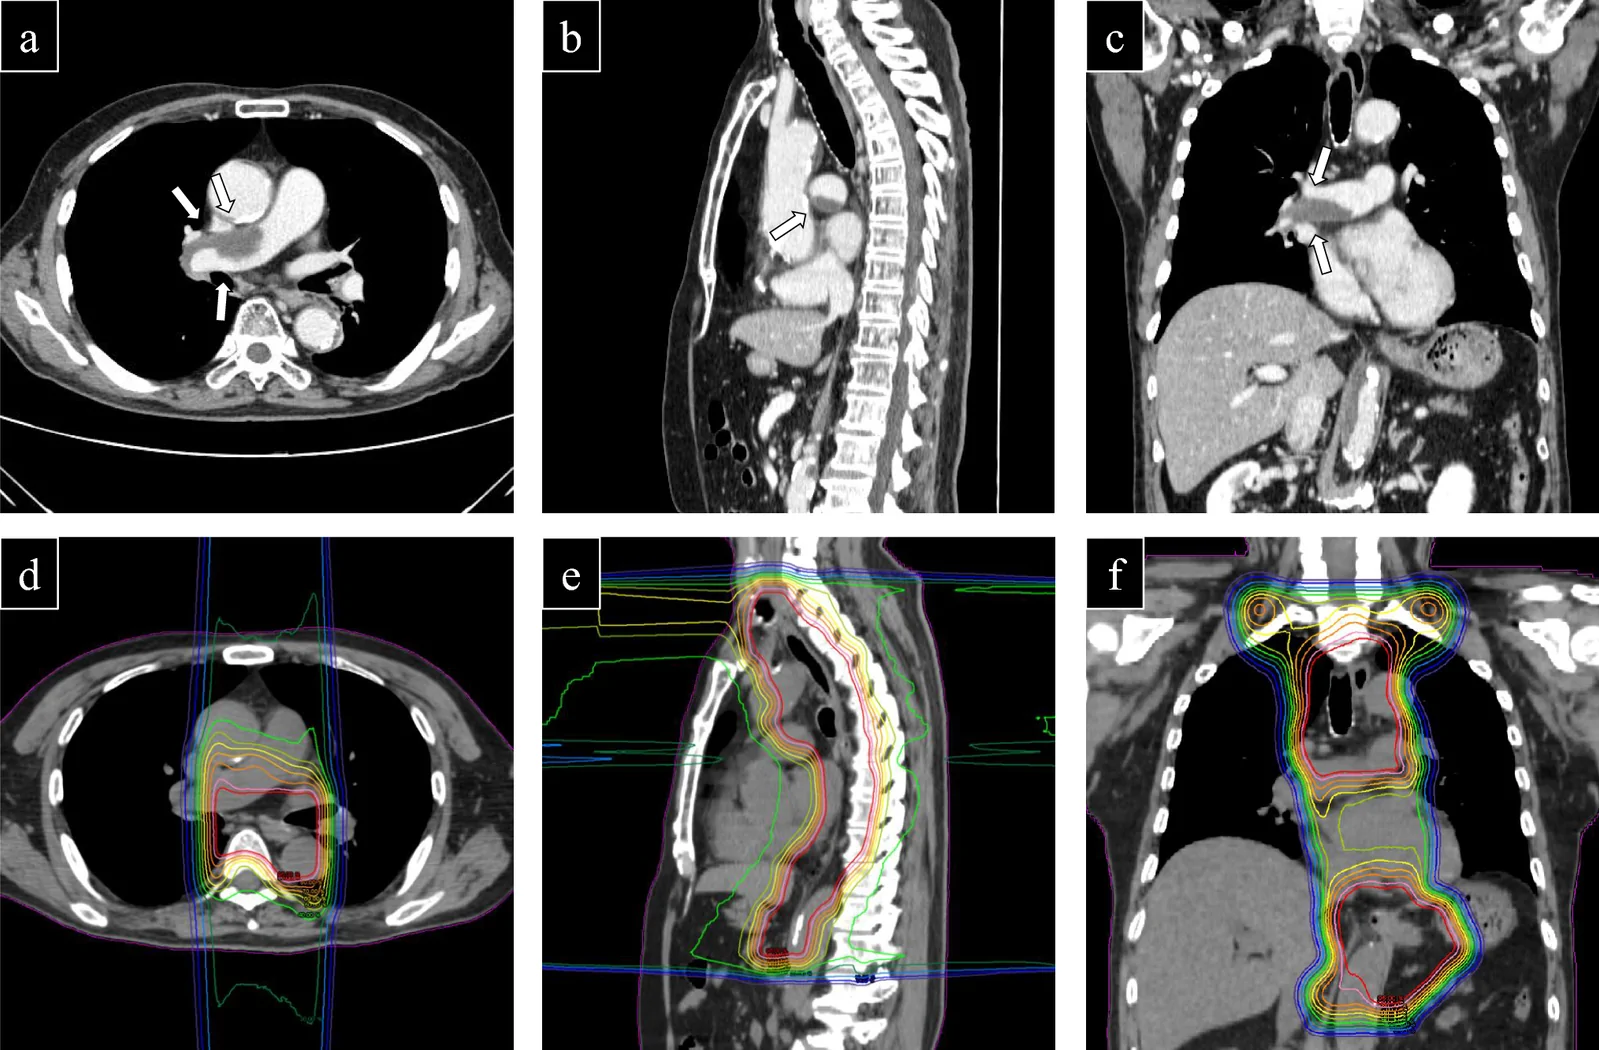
In situ pulmonary artery thrombosis after proton beam therapy. a-c Contrast-enhanced CT images obtained 32 months after proton beam therapy (PBT) for esophageal cancer. Axial (a), sagittal (b), and coronal (c) views demonstrate a large, eccentric filling defect within the right pulmonary artery without contrast enhancement (arrows). d-f Corresponding CT images overlaid with PBT dose distribution (d: axial, e: sagittal, f: coronal). The isodose lines are shown in rainbow colors, with the red line representing the 95% prescribed dose. The filling defect in the right pulmonary artery corresponds to the mediastinal irradiation field
